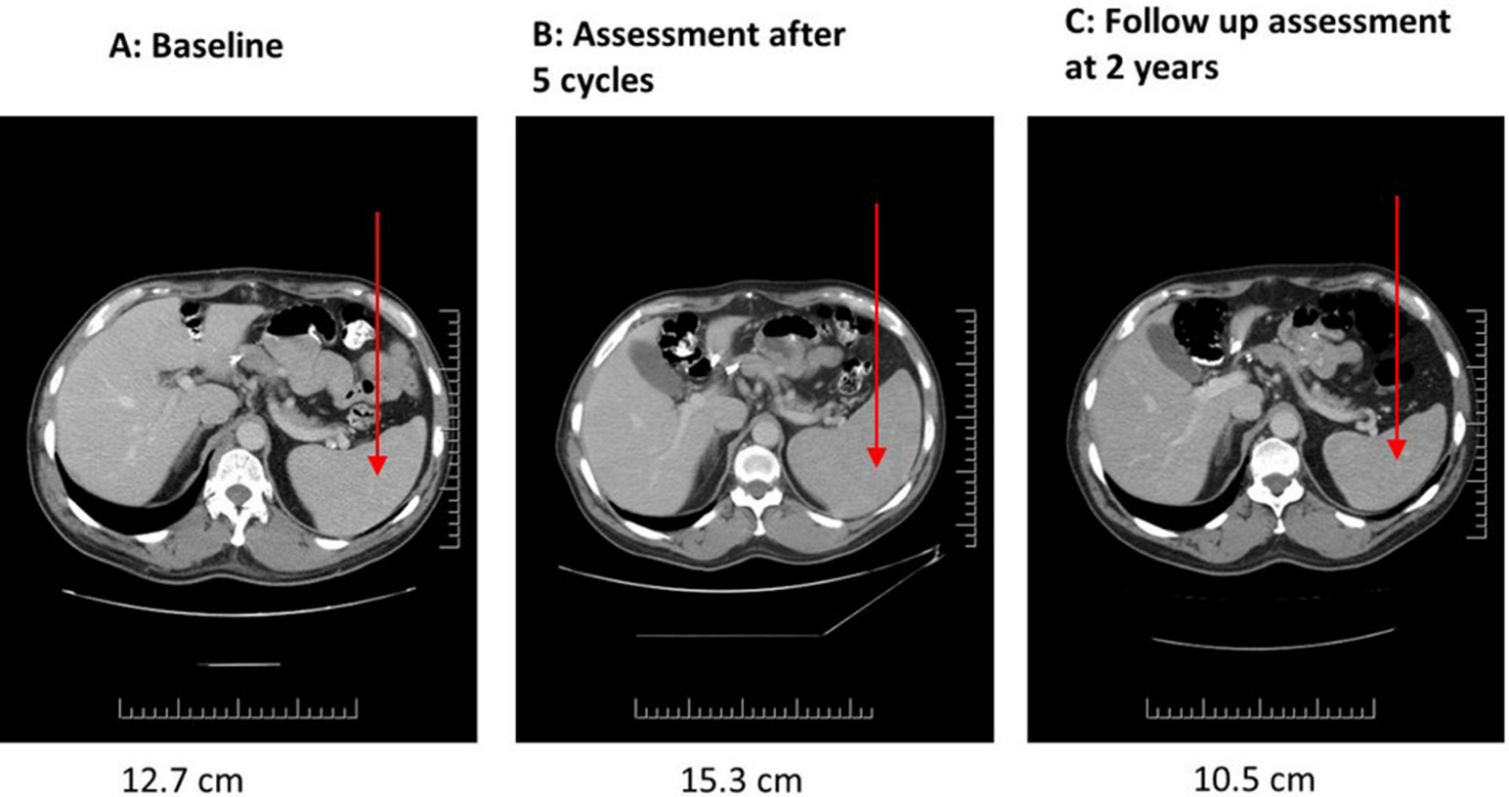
Patient 03 serial CT scans showing appearance and progression of splenomegaly at baseline (A, 12.7 cm), post 5 cycles of NK-92 therapy (B, 15.3 cm) and long-term follow-up at two years post-treatment (C, 10.5 cm).
